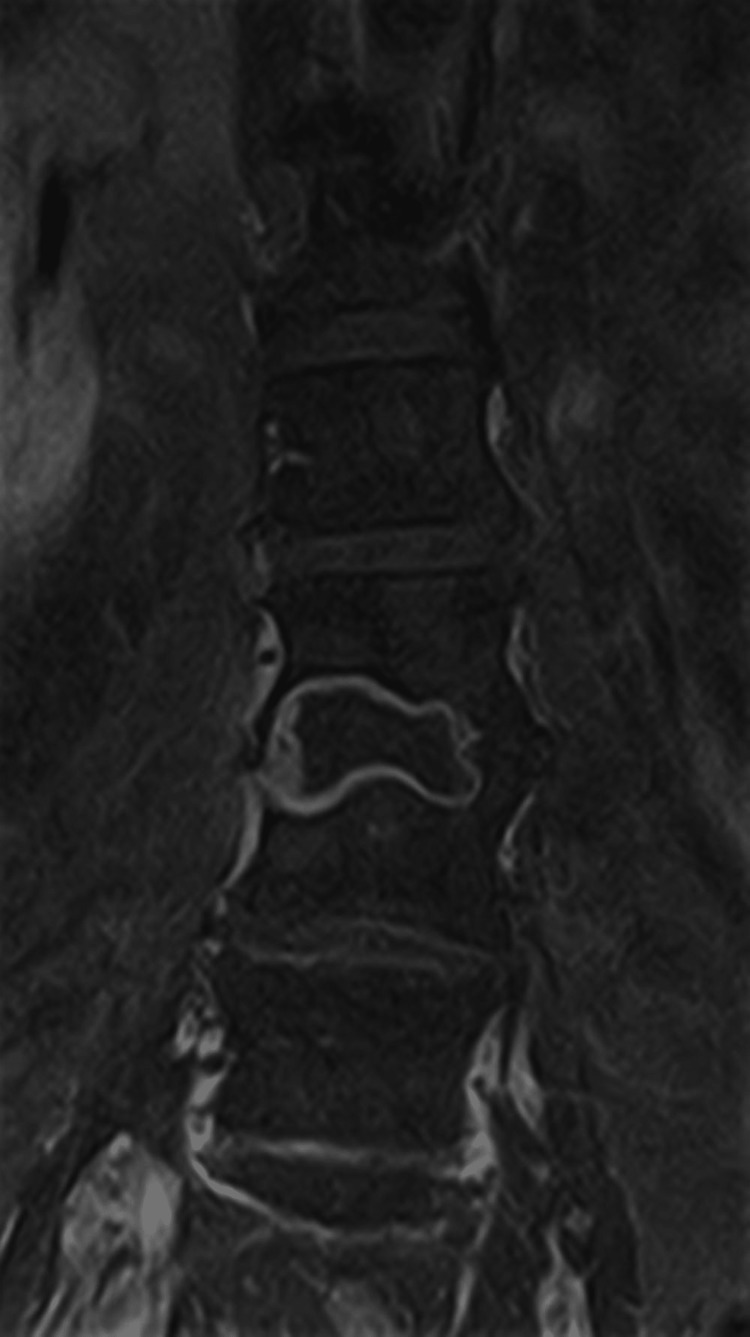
Last control MRI, 11 months after the beginning of the treatment (coronal view)
